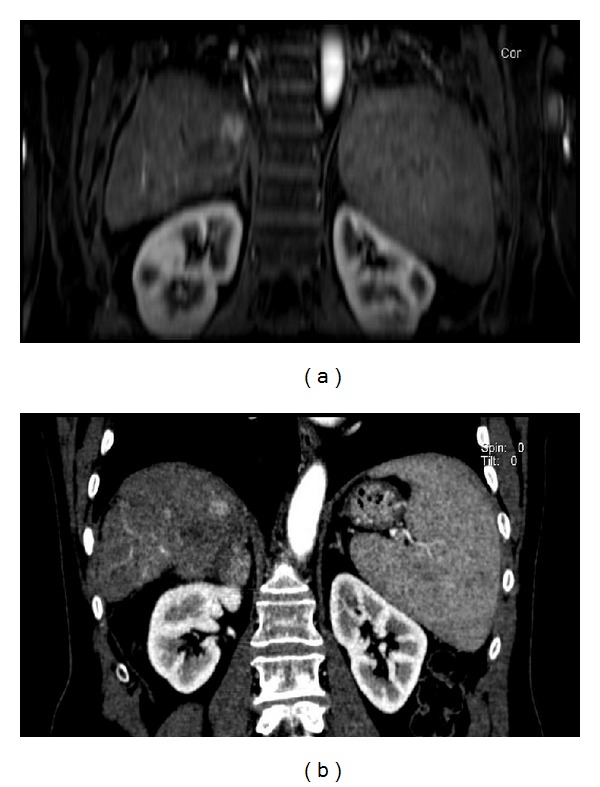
77-year-old man treated with RFA for HCC. (a) Arterial-phase, coronal Vibe T1-W FS at 1-month followup. Residual tumor tissue in the cephalad portion of the treated area. (b) Coronal reformatted, artery-phase CT at 1-month followup. Residual tumor tissue in the cephalad portion of the treated area.
